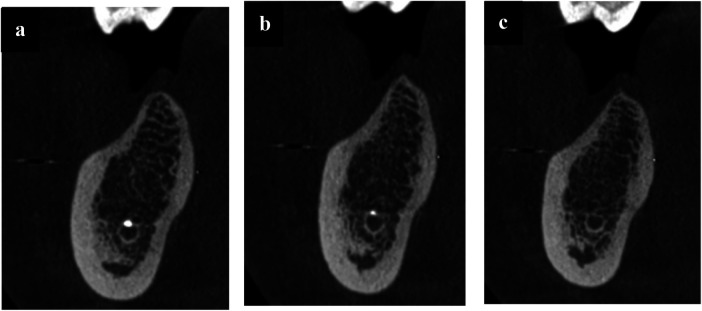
(a–c) sagittal CBCT sections taken after three years of follow-up, showing IAN canal with incomplete canal obturation.
